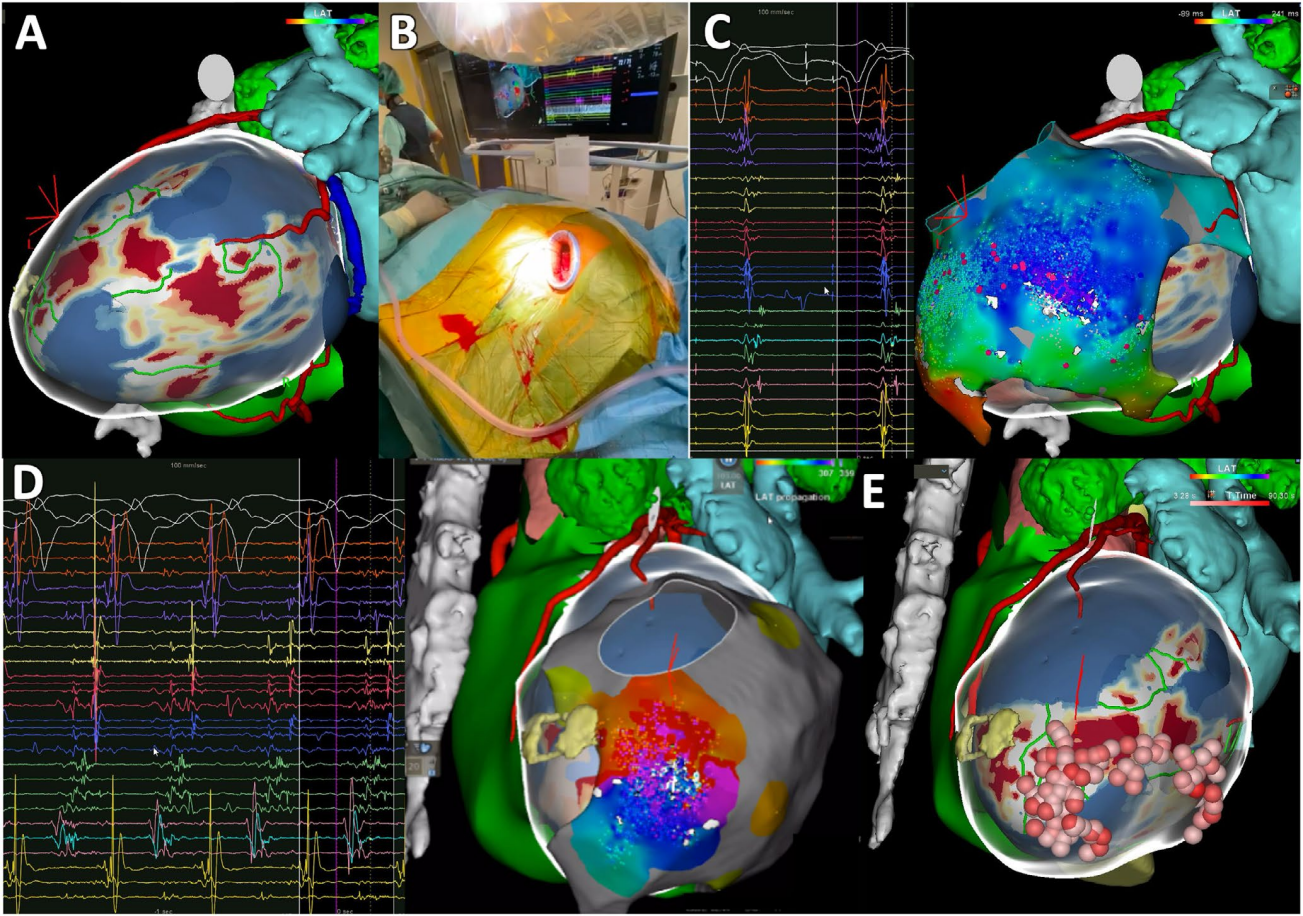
Ventricular tachycardia ablation in a 59‐year‐old male patient with valvular heart disease, with mechanical aortic and mitral prostheses, inaccessible endocardium, and extensive epicardial adhesions. As LGE‐CMR predicted arrhythmogenic channels in thin areas of the apical and mid‐ventricular segments of the lateral wall, an epicardial approach through a left lateral mini‐thoracotomy with localized manual debridement of epicardial adhesions was considered a viable treatment option. (A) ADAS 3D segmentation of LGE‐CMR depicting core scar and border zones in the apical and mid‐ventricular segments of the lateral wall, with several predicted intra‐scar corridors involving the subendocardial, mid‐mural, and subepicardial layers (green lines). Remaining anatomic structures reconstructed from MDCT include the left atrium (light blue), aorta (light red), left coronary artery (red), coronary sinus (dark blue), RV and pulmonary artery (green), and sternum (grey). (B) Left lateral mini‐thoracotomy targeting the predicted VT isthmus location. (C) Substrate activation map obtained during RV pacing with LAVA detected in the predicted area of interest. (D) VT activation map documenting the protected isthmus at a location corresponding to a predicted intra‐scar corridor. (E) Final ablation setting with unipolar RF epicardial applications. The patient remains free from arrhythmic events without medication, with a follow‐up duration now exceeding 18 months. 3D, Tridimensional; LAVA, Local abnormal ventricular activity; LGE‐CMR, Late gadolinium enhancement cardiac magnetic resonance; MDCT, Multidetector computed tomography; RF, Radiofrequency; RV, Right ventricle; VT, Ventricular tachycardia.
